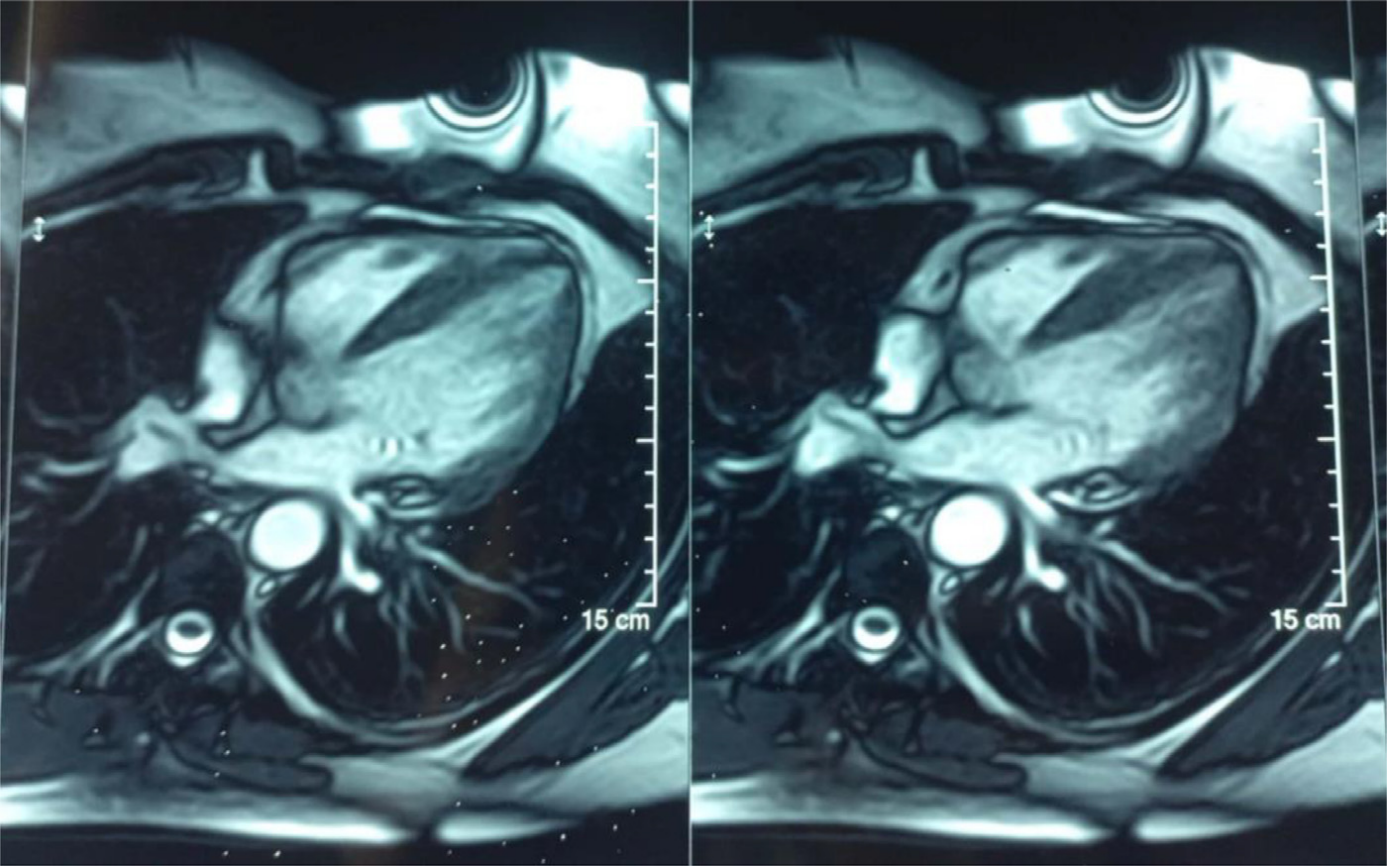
Cardiac MRI 4-chamber sequence showing late subepicardial enhancement in the middle and apical segments of the lateral wall.
